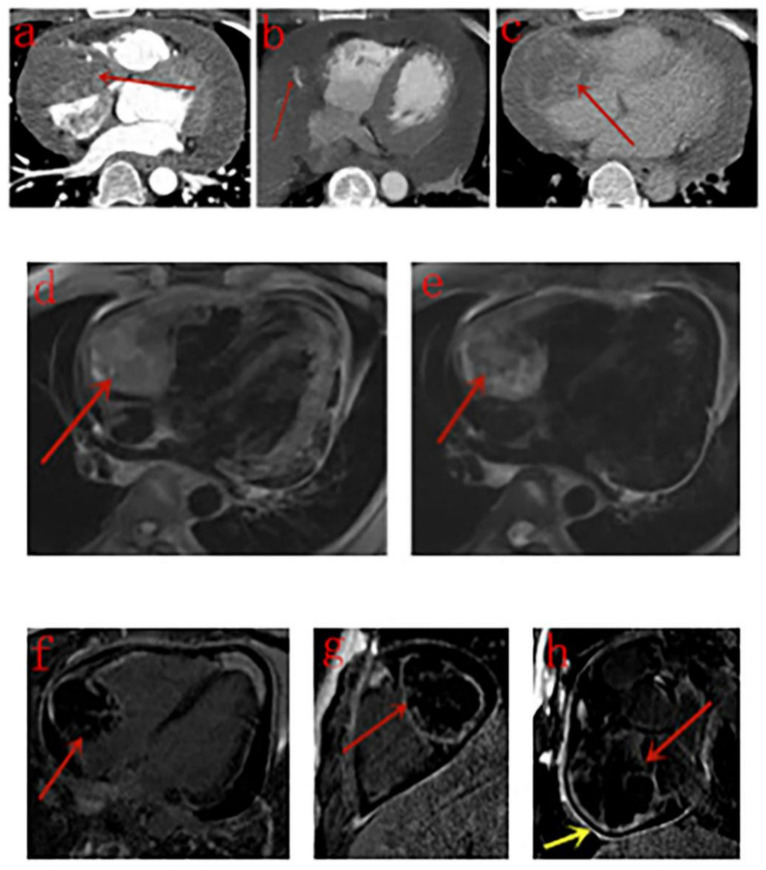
Male, 34 years old, right atrium angiosarcoma. (a–c): The mass (red arrow) is heterogeneously enhanced in the arterial (a) and venous (c) phase of contrast-enhanced CT images, and small tortuous vessels (b, red arrow) deriving from the right coronary artery (RCA) and pericardial effusion are noticed. (d,e): The mass (red arrow) presents as heterogeneous intensity, with multiple hyperintense hemorrhage foci on T1WI (d) and T2WI (e). (f–h): late-phase gadolinium enhancement (LGE) shows heterogeneous rim enhancement (red arrow) on four-chamber (f), two-chamber (g), and short axis (h) planes. Pericardium enhancement is noticed in the short axis plane (yellow arrow).
